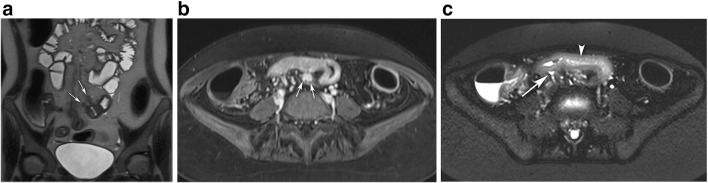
MRI of a 21-year-old man with Crohn’s disease, abdominal pain, and diarrhea. a Coronal T2-weighted image shows two sinus tracts of the ileum (arrows) with a characteristic mesenteric orientation within a stenotic bowel segment. On post-contrast T1-weighted fat-saturated imaging (b), marked contrast enhancement can be seen (arrows). Axial T2-weighted fat-saturated image (c) shows mural edema (arrowhead) and perienteral fluid rim (arrow), resulting in a CDMI score of 10. The patient underwent subsequent surgery with resection of the ileal segment
